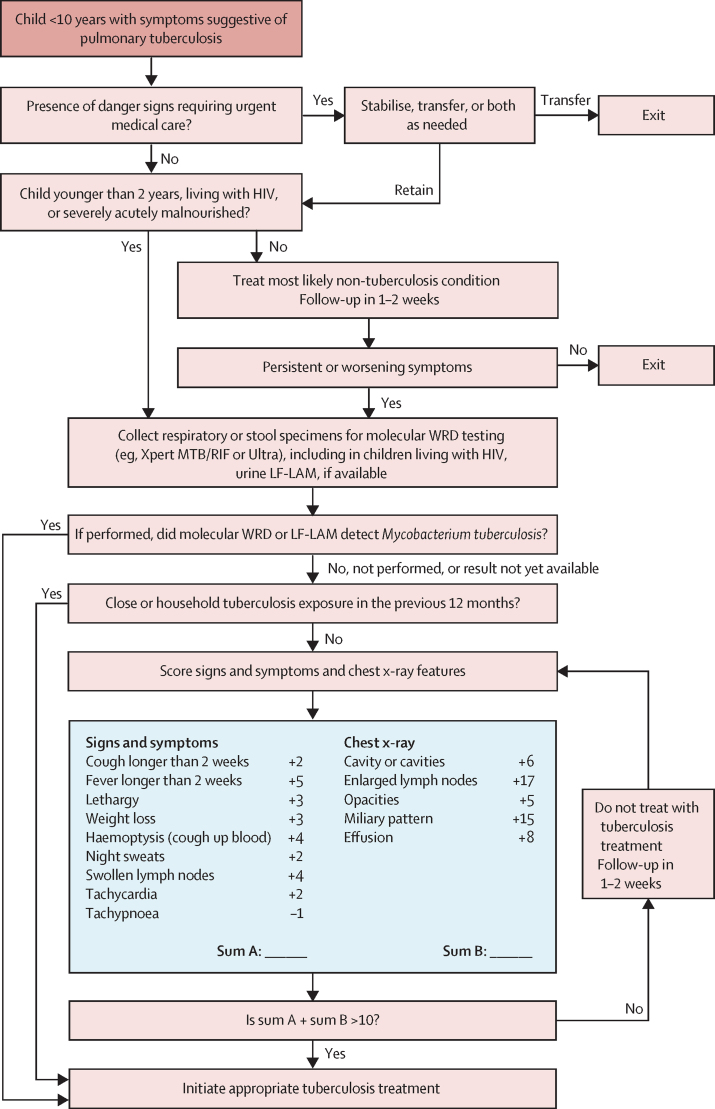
Treatment-decision algorithm including chest x-ray features derived from the prediction model Tuberculosis treatment-decision algorithm for use among children younger than 10 years with symptoms suggestive of pulmonary tuberculosis, reproduced from the operational handbook accompanying the WHO consolidated guidelines on the management of tuberculosis in children and adolescents.^12^, ^13^ Selection steps before entering the scoring system reflect recommendations from the WHO expert panel to enrich the probability of tuberculosis among the population of children proceeding through the algorithm to the model such that the probability would more closely reflect the preselected population producing the data from which the prediction model was built, while balancing the consequences of untreated tuberculosis in children at high risk. Scores associated with features from clinical history and physical exam and chest x-ray translate to risk of tuberculosis and are scaled from the prediction model developed from the individual participant dataset. Guidance on the practical use of this algorithm is outlined in the WHO operational handbook.^13^ LF-LAM=lateral flow urine lipoarabinomannan assay. WRD=WHO-recommended rapid diagnostic test.
